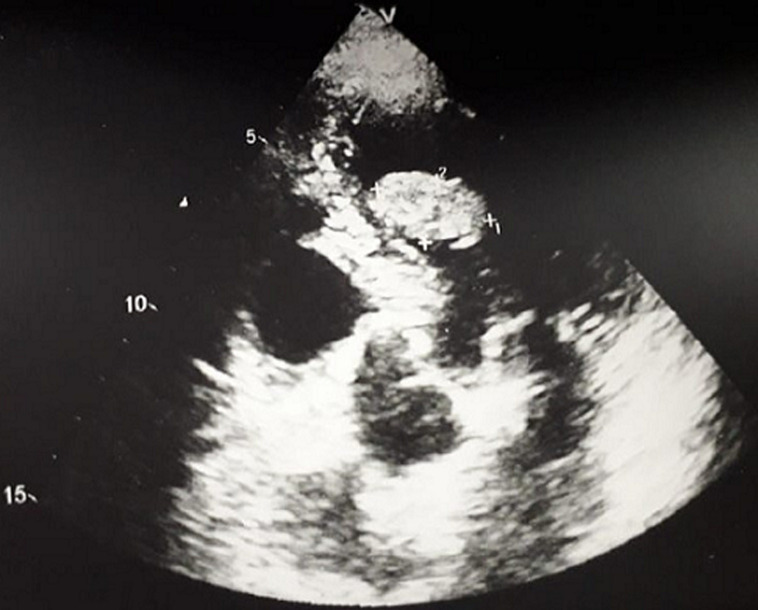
echocardiogram demonstrating a left ventricular mass in a 62-year-old asymptomatic male patient
